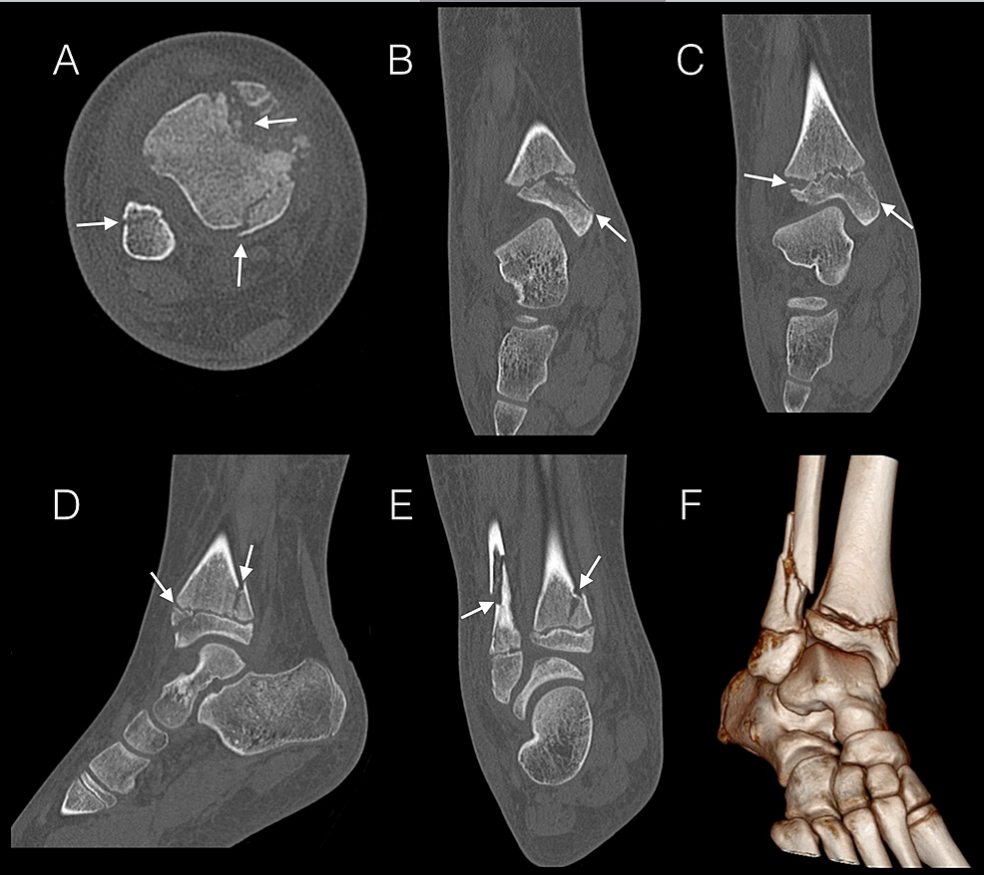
CT of the right ankle. A: Axial reconstruction showing the spiral fracture of the fibula, posterior malleolar fracture, and anteromedial avulsion fracture of the medial malleolus. B: Coronal reconstruction demonstrating the extra-articular anteromedial epiphyseal sleeve avulsion of the medial malleolus. C: Coronal reconstruction demonstrating anterolateral physeal widening and the medial malleolar fracture. D: Sagittal reconstruction demonstrating both the posterior malleolar and extra-articular medial malleolar fractures. E: Coronal reconstruction capturing the posterior malleolar fracture and spiral fracture of the fibula. F: Volume rendered three-dimensional reconstruction.
